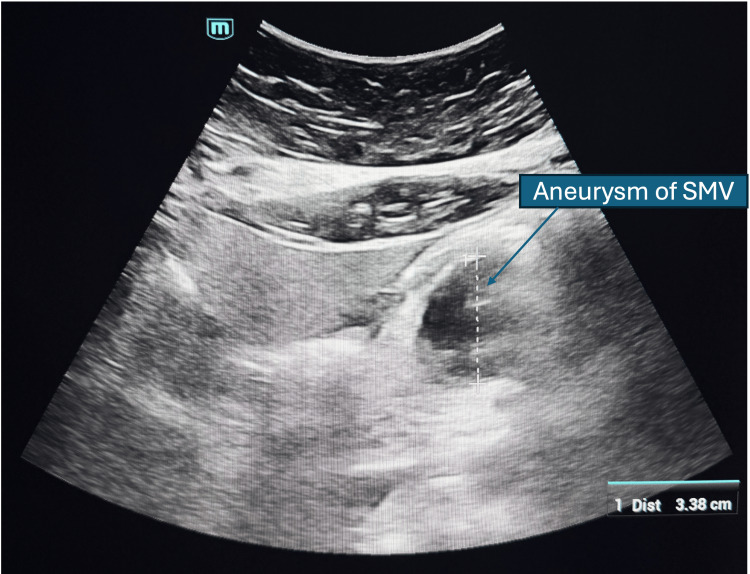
Serial Doppler ultrasound follow-up of the superior mesenteric vein (SMV) aneurysmal lesion and associated thrombosis under immunosuppressive therapy and anticoagulation, demonstrating progressive reduction in lesion diameter and partial portal vein recanalization. Baseline Doppler evaluation showed the absence of detectable flow within the aneurysmal sac.
